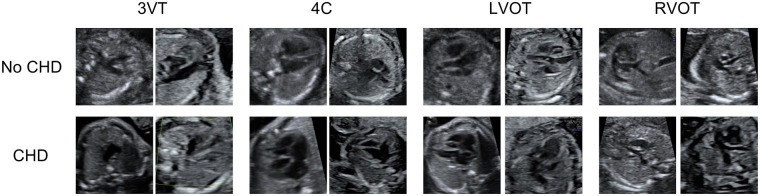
Examples of echocardiographic views from Heartbeat. Representative images of Congenital Heart Disease (CHD) and non-CHD cases are shown for the three-vessel trachea (3VT), four-chamber (4C), left ventricular outflow tract (LVOT), and right ventricular outflow tract (RVOT) views. Each view offers complementary anatomical information that is essential for accurate CHD detection.
